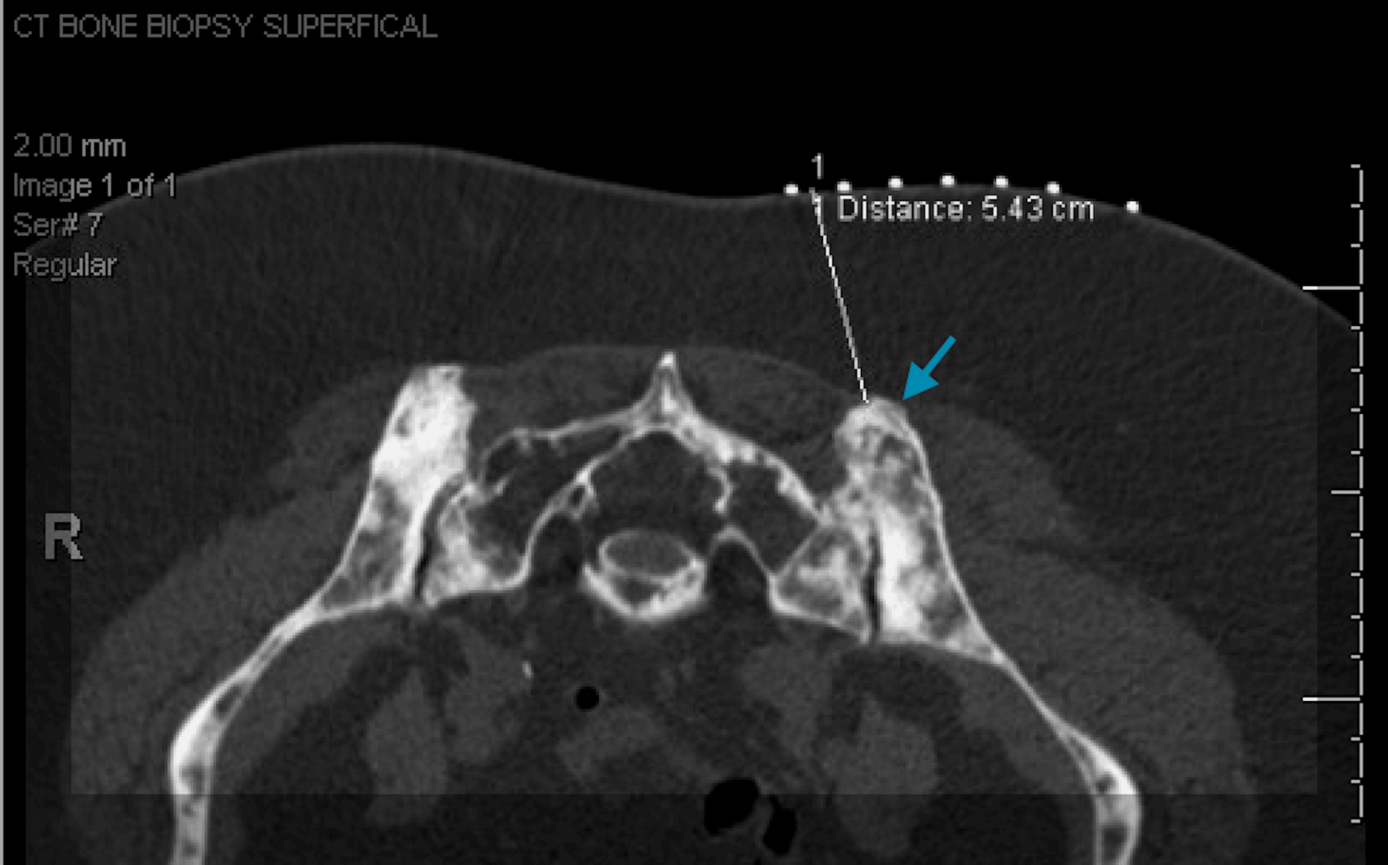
Osseous lesions identified on CT scan. Imaging from the CT-guided targeted biopsy of lytic lesions in the left posterior ischial spine (blue arrow).
